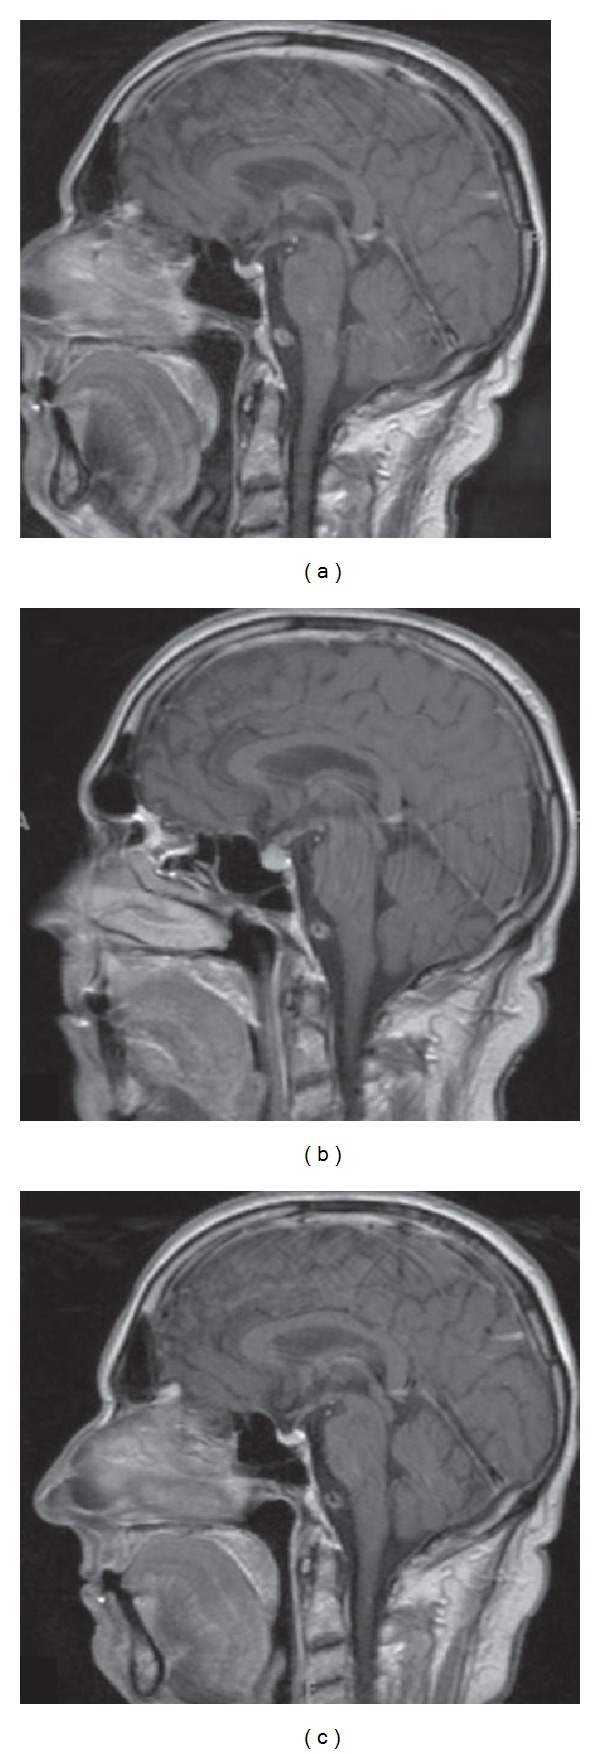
Magnetic resonance images of the brain demonstrating ipilimumab-associated hypophysitis. (a) Prior to therapy, with no metastatic disease indicated. (b) Diffuse enlargement of the pituitary gland following reports of cognitive impairment during therapy. (c) Resolution of hypophysitis after discontinuation of ipilimumab and initiation of hormone-replacement therapy [14]; reproduced with permission from Carpenter et al. 2009 [14].
